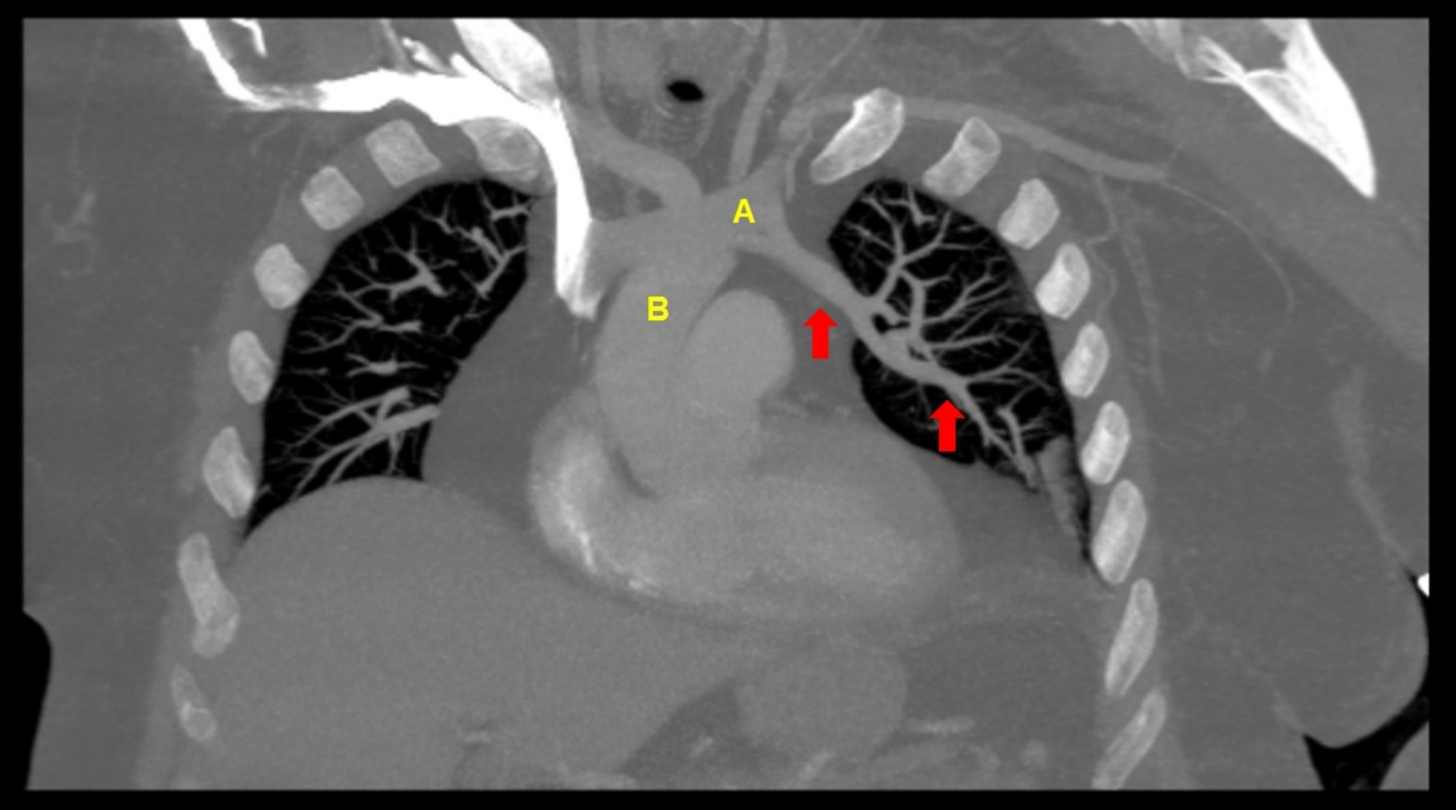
CT-angiogram coronal plan showing PAPVC A CT-angiogram of the pulmonary arteries, coronal plan, revealing the incidental finding of a left partial anomalous pulmonary venous return (red arrows), returning blood to the left brachiocephalic vein (A), which leads to the superior vena cava (B) and the right atrium.
